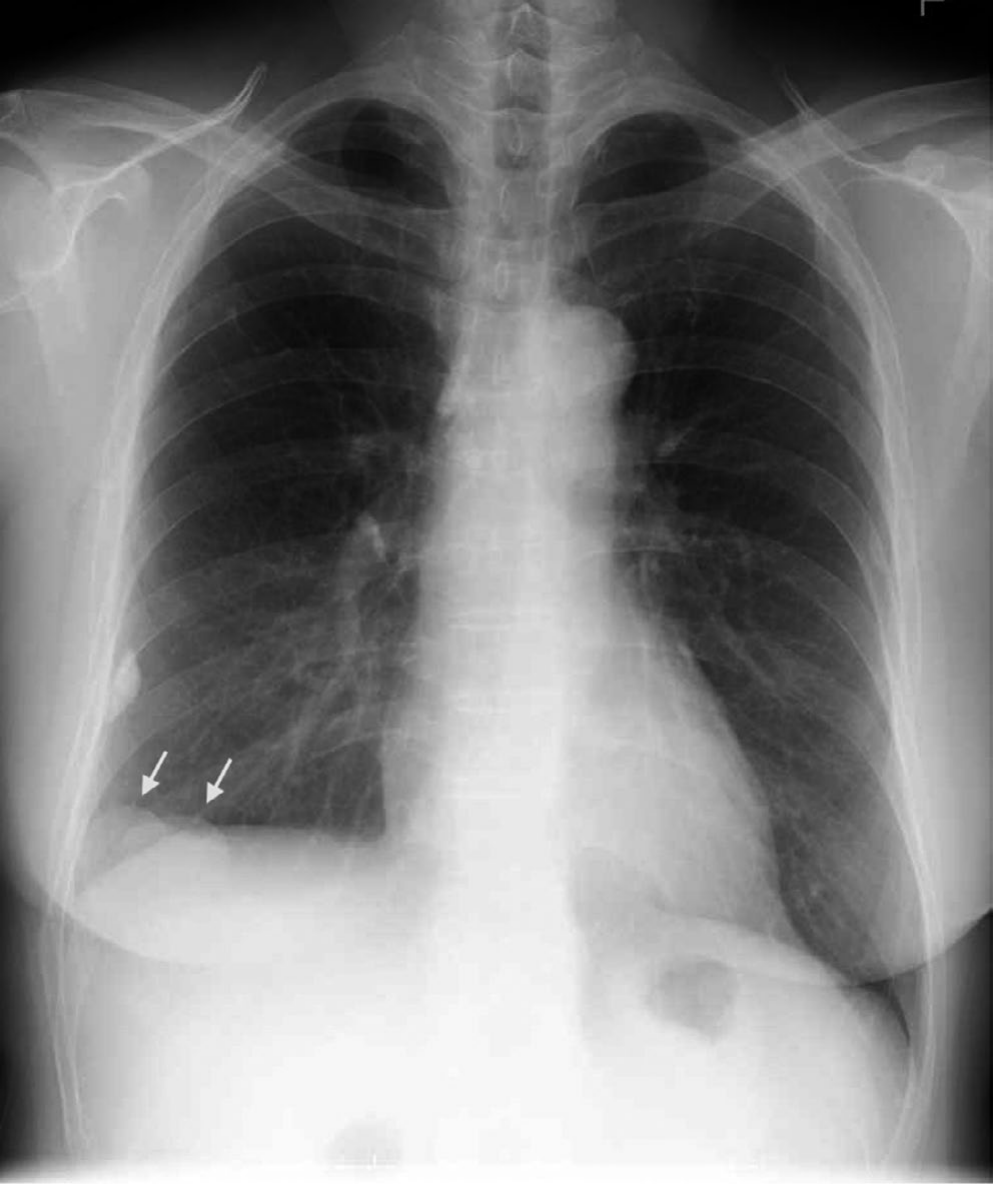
Chest radiography showed two masses in the lower field and a calcified nodule in the middle field of the right lung. Arrows indicate liver herniations
